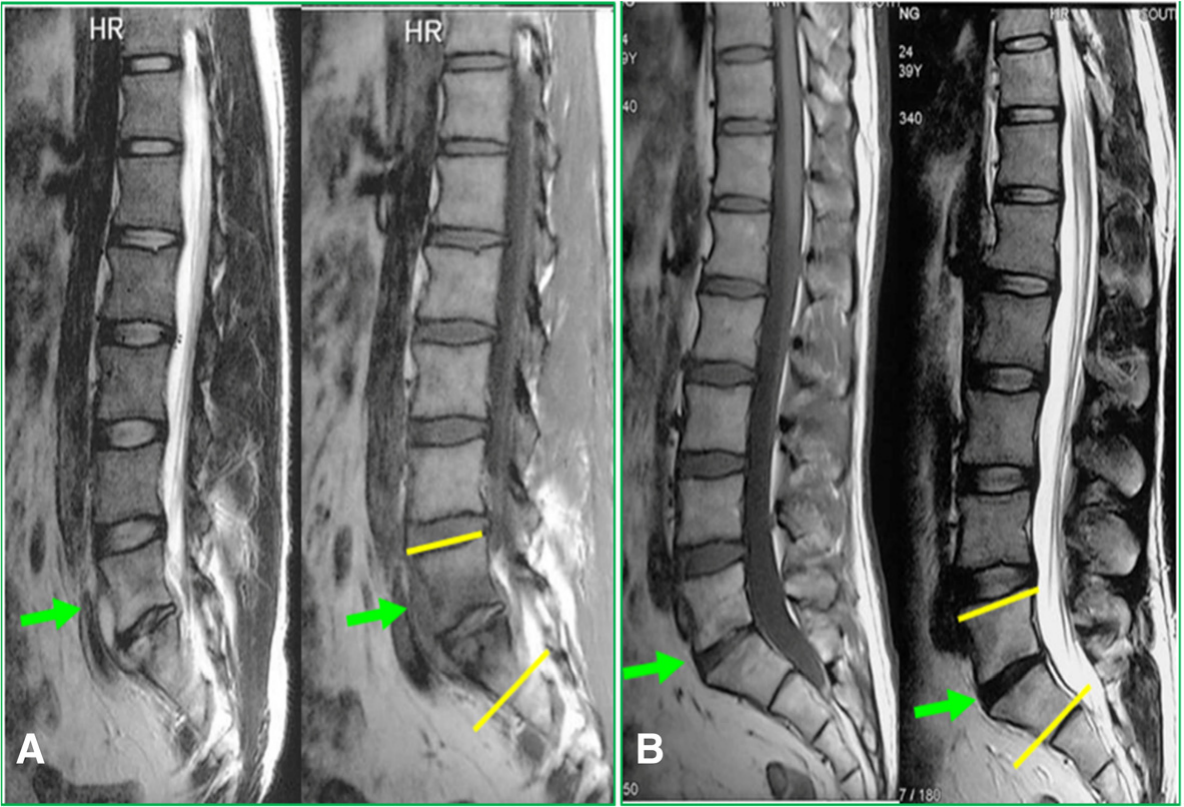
This is a 34-year-old male who was diagnosed of mild spinal tuberculosis (L5/S1) with sacral abscess; the patient was treated with standard chemotherapy for 18 months. a The MRI before the treatment shows an intervertebral edema of L5/S1 and the formation of sacral abscess anteriorly (*arrow*); the Cobb angle of local lordosis (*yellow lines*) was 33.4°. b The MRI after 5 years shows complete absence of sacral abscess and the intervertebral edema, and the Cobb angle of local lordosis (*yellow lines*) was 31.5°; there was no significant progression of local kyphosis or simultaneous spinal fusion
